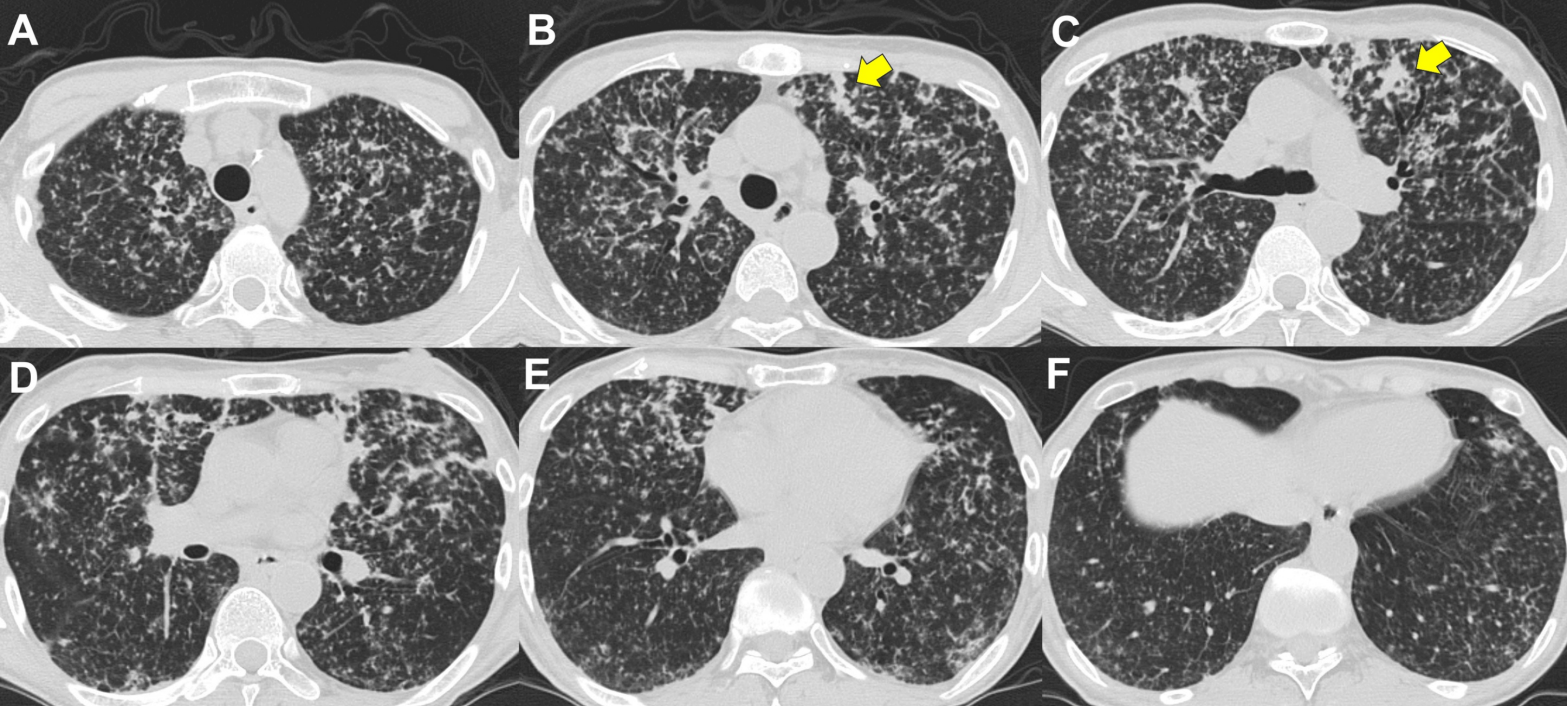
Chest computed tomography Axial thin-section computed tomography images (slice thickness, 0.6 mm; interslice gap, 0.6 mm) demonstrate diffuse centrilobular nodules predominantly involving the upper to middle lung zones of both lungs, corresponding to the reticulonodular opacities observed on the chest radiograph. Some of the nodules show partial confluence (yellow arrows in B and C). Nodules distributed along the bronchovascular bundles are also noted (yellow arrow in B), raising lymphatic involvement as a differential consideration. No focal mass lesions, lobar consolidation, or significant lymphadenopathy are present.
